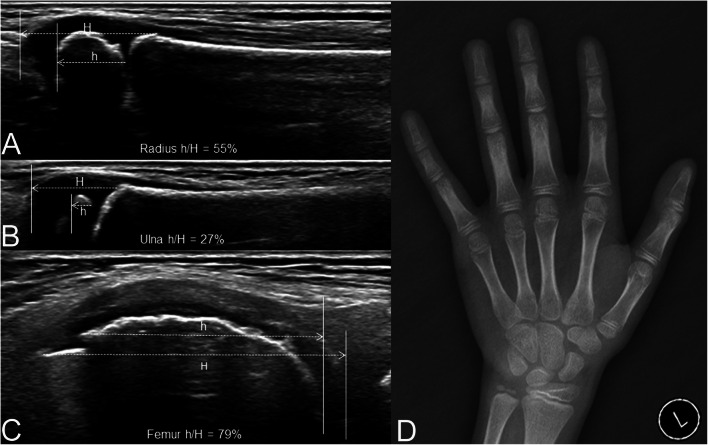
Ultrasound images of the distal end of the left radius (A), ulna (B), and femur (C) and the X-ray film of left hand and wrist (D) in a 10.9-year-old Chinese boy with complaint of precocious puberty
